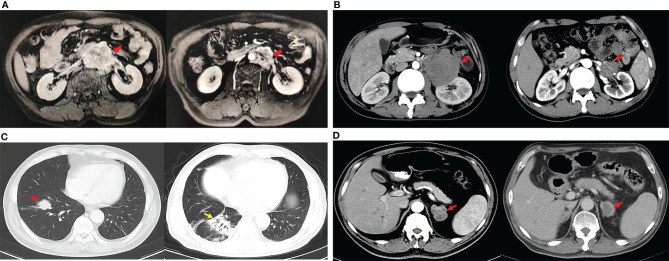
Changes of metastatic lesions during treatments. (A). PR of the patient after first-line chemotherapy (paclitaxel and cisplatin): the maximum diameter of the retroperitoneal lymph node metastasis (red arrows) decreased from 7.4 cm (left) to 4.4 cm (right). (B) The enhanced CT showed the shrinkage of the retroperitoneal metastasis (red arrows) after sintilimab plus anlotinib. The maximum diameter of the lesion decreased from 8.2 cm (left) to 2.6 cm (right) considering partial response. (C) The enhanced chest CT showed the metastasis located at the right lung (red arrow) disappeared after PD-1 antibody, but interstitial pneumonia appeared (yellow arrow). (D) Meanwhile, the maximum diameter of the left adrenal metastasis (red arrows) decreased from 3.6 cm (left) to 2.8 cm (right) of the same patient described in (C), so the partial response was considered.
